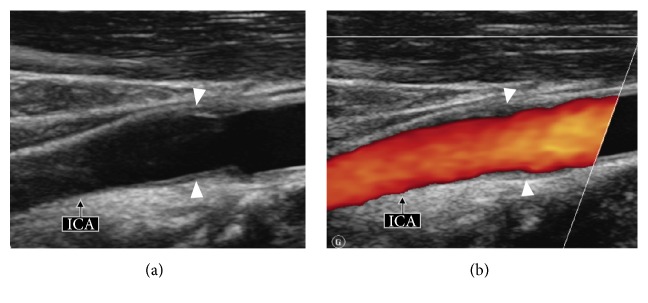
US longitudinal view (a) and corresponding color Doppler image (b) obtained 4 months later show regression of the fusiform wall thickening (arrowheads) of the left carotid bulb. ICA: internal carotid artery. See for comparison Figures 1(b) and 1(c) obtained 4 months earlier.
